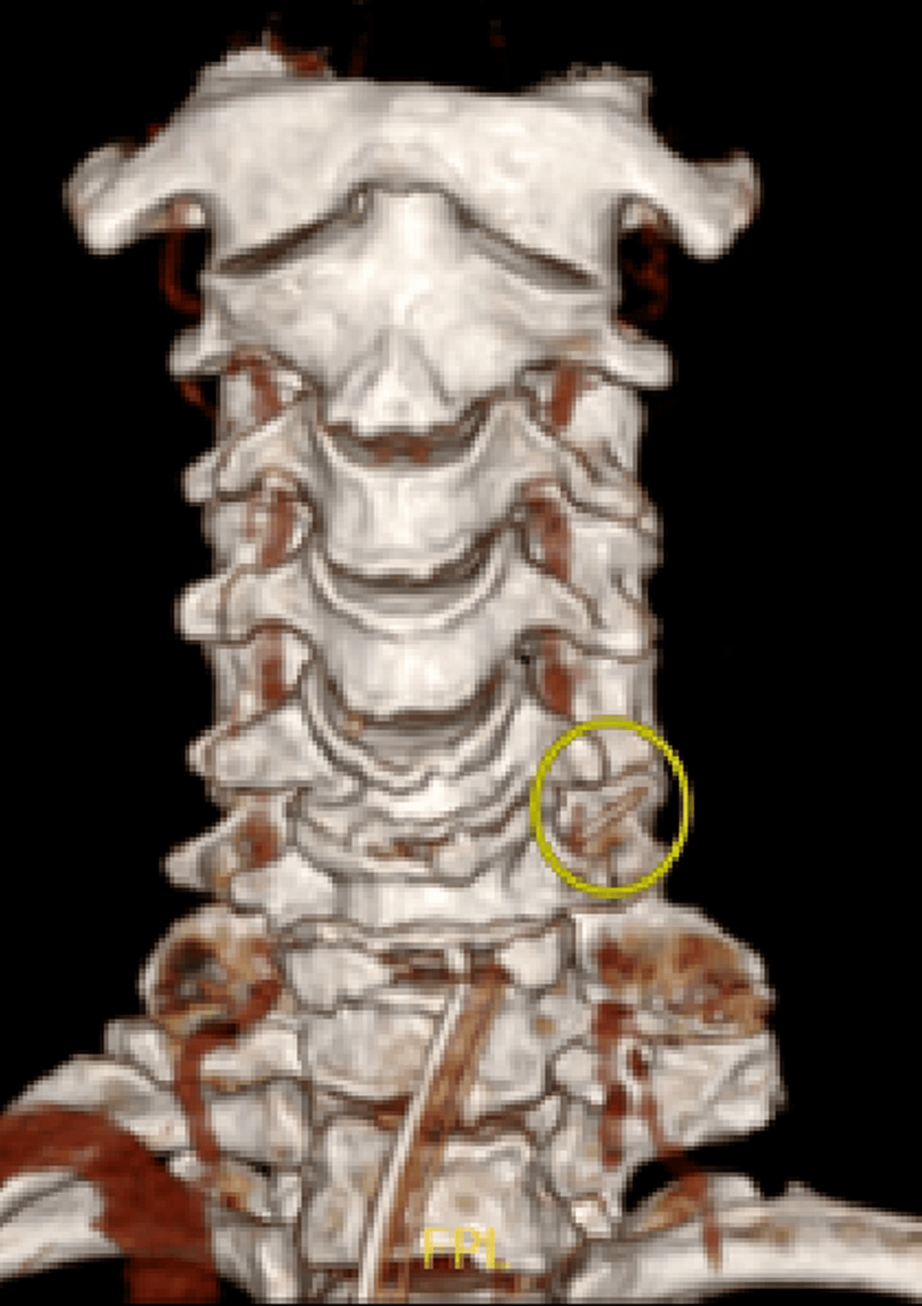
CT cervical (coronal view) of the patient in three-dimensional reconstruction showing the foreign body in the marked area
